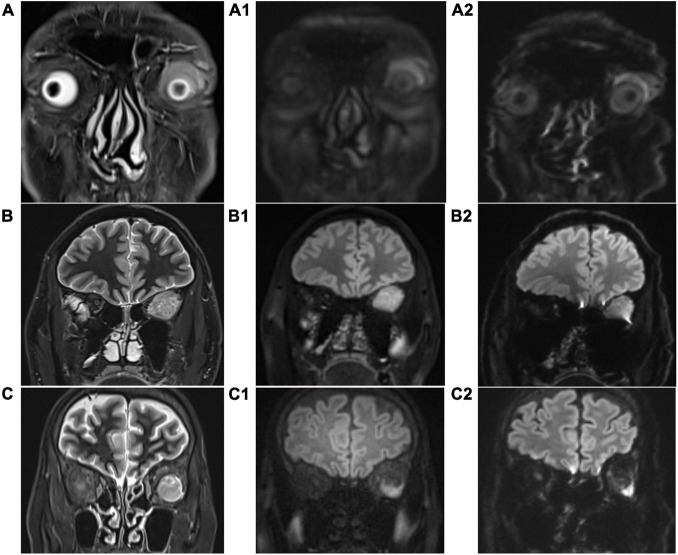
Coronal T2-weighted imaging (T2WI) images (A–C), coronal TGSE-PROPELLER-DWI b1000 images (A1–C1), and coronal rs-EPI b1000 images (A2–C2). (A,A1,A2): patient 1, male, 62 years old, left orbital lymphoma, scores of geometric distortions, susceptibility artifacts, lesion conspicuity, and overall image quality of TGSE-BLADE-DWI were 4, 4, 4, and 4, respectively, while those of rs-EPI were 3, 2, 3, and 3, respectively. (B,B1,B2): patient 2, male, 50 years old, left orbital fibroma, scores of geometric distortions, susceptibility artifacts, lesion conspicuity, and overall image quality of TGSE-BLADE-DWI were 4, 4, 4, and 4, respectively, while those of rs-EPI were 3, 3, 4, and 4, respectively. (C,C1,C2): patient 3, female, 61 years old, left orbital hemangioma, scores of geometric distortions, susceptibility artifacts, lesion conspicuity, and overall image quality of TGSE-BLADE-DWI were 4, 4, 4, and 4, respectively, while those of rs-EPI were 2, 3, 2, and 2, respectively.
